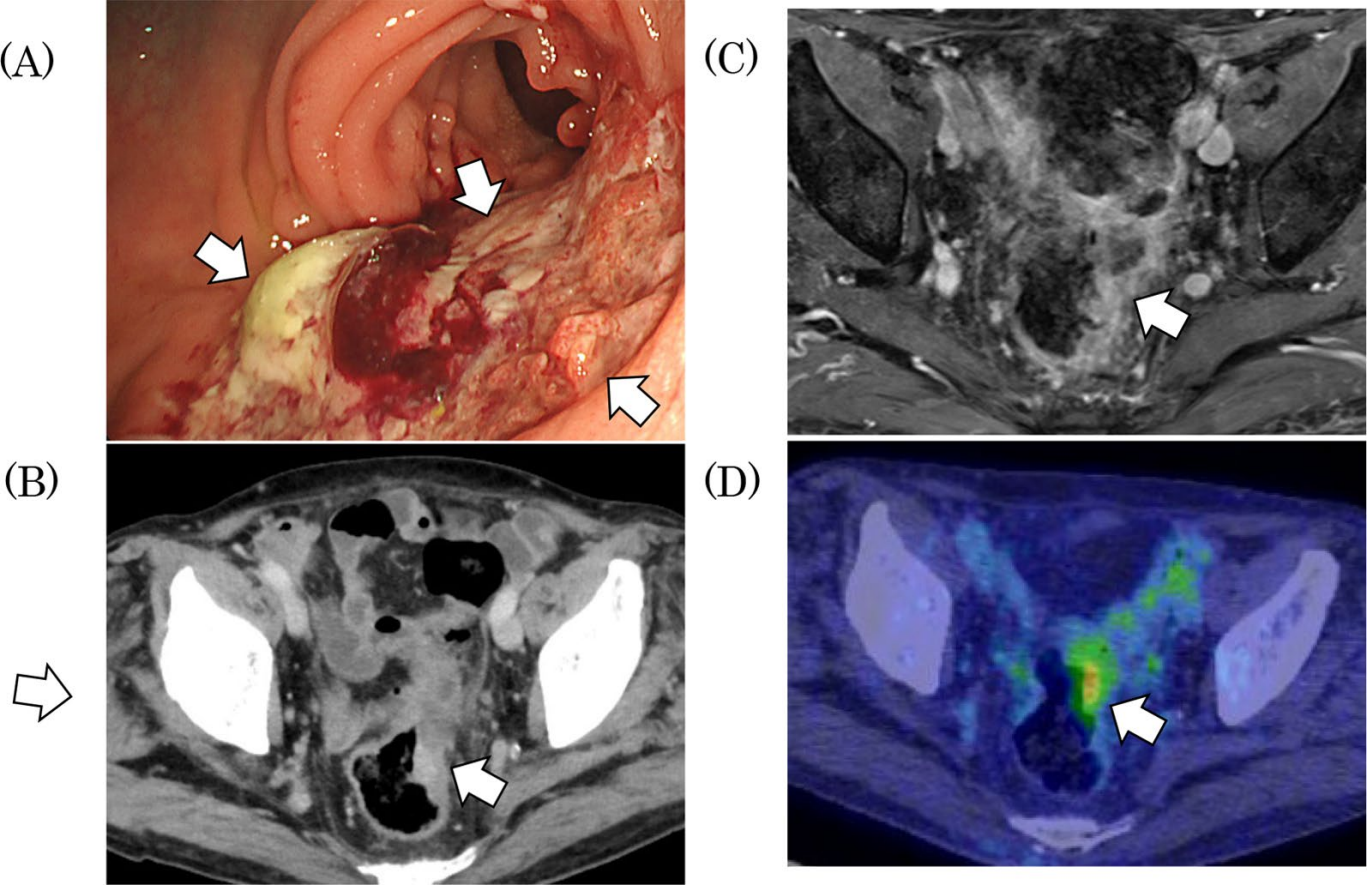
Imaging conducted after treatment with neoadjuvant mFOLFOX6 followed by ipilimumab and nivolumab in Case 1. A Endoscopic imaging showing a sigmoid colon cancer turned ulcer. B CT scan, after ipilimumab and nivolumab, showing the tumor’s notable shrinkage. C Magnetic resonance imaging (MRI) after ipilimumab and nivolumab therapy, showing the tumor’s notable shrinking. D Positron emission tomography (PET)–CT after ipilimumab and nivolumab therapy, showing the existence of tumor hot spots. Arrows identify the tumor
